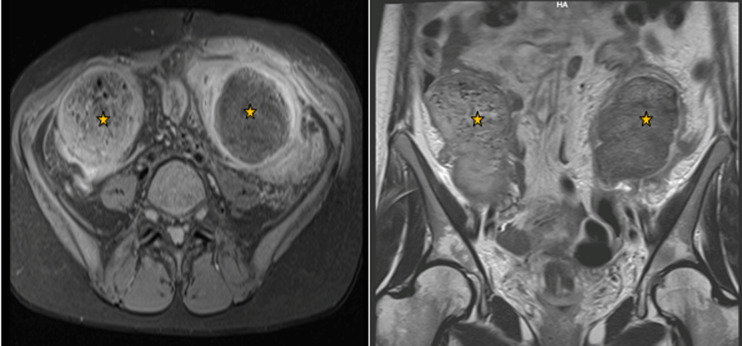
MRI views showing two well-circumscribed collections located in the right and left iliac fossa with spongiform pattern and entrapped air bubbles
